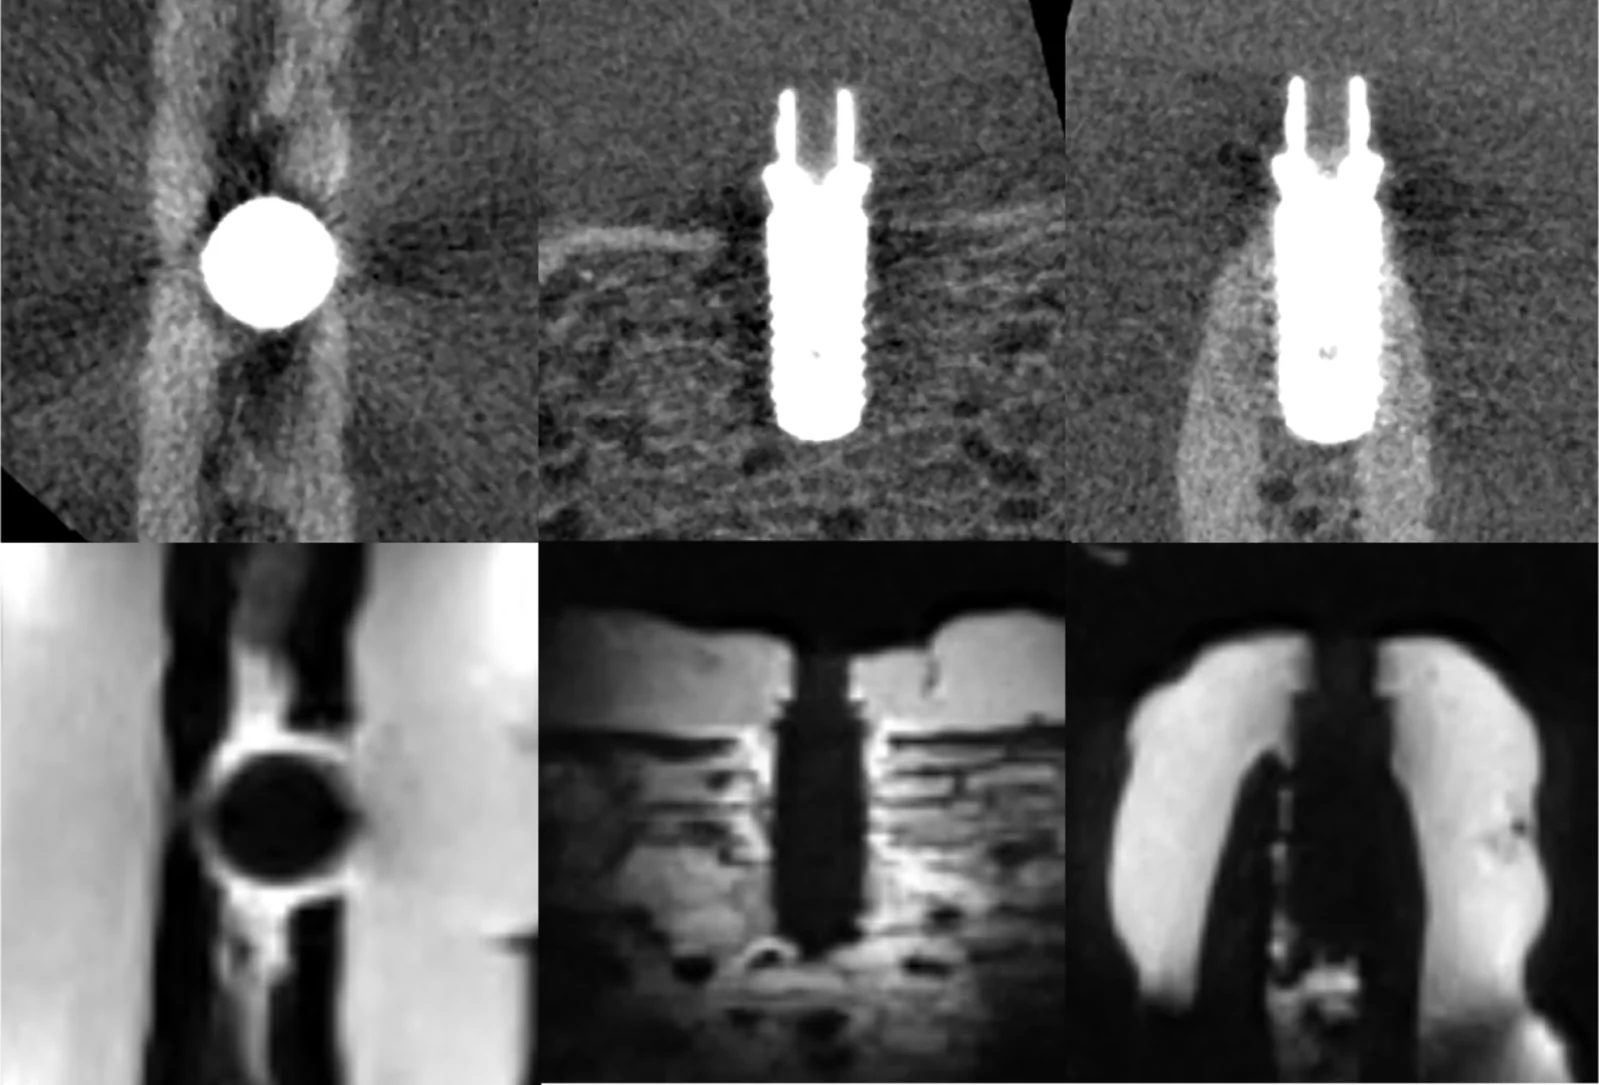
Examples of small 3-wall defects in CBCT/dMRI (upper/lower row) in axial, sagittal, and coronal reconstruction (left to right)
